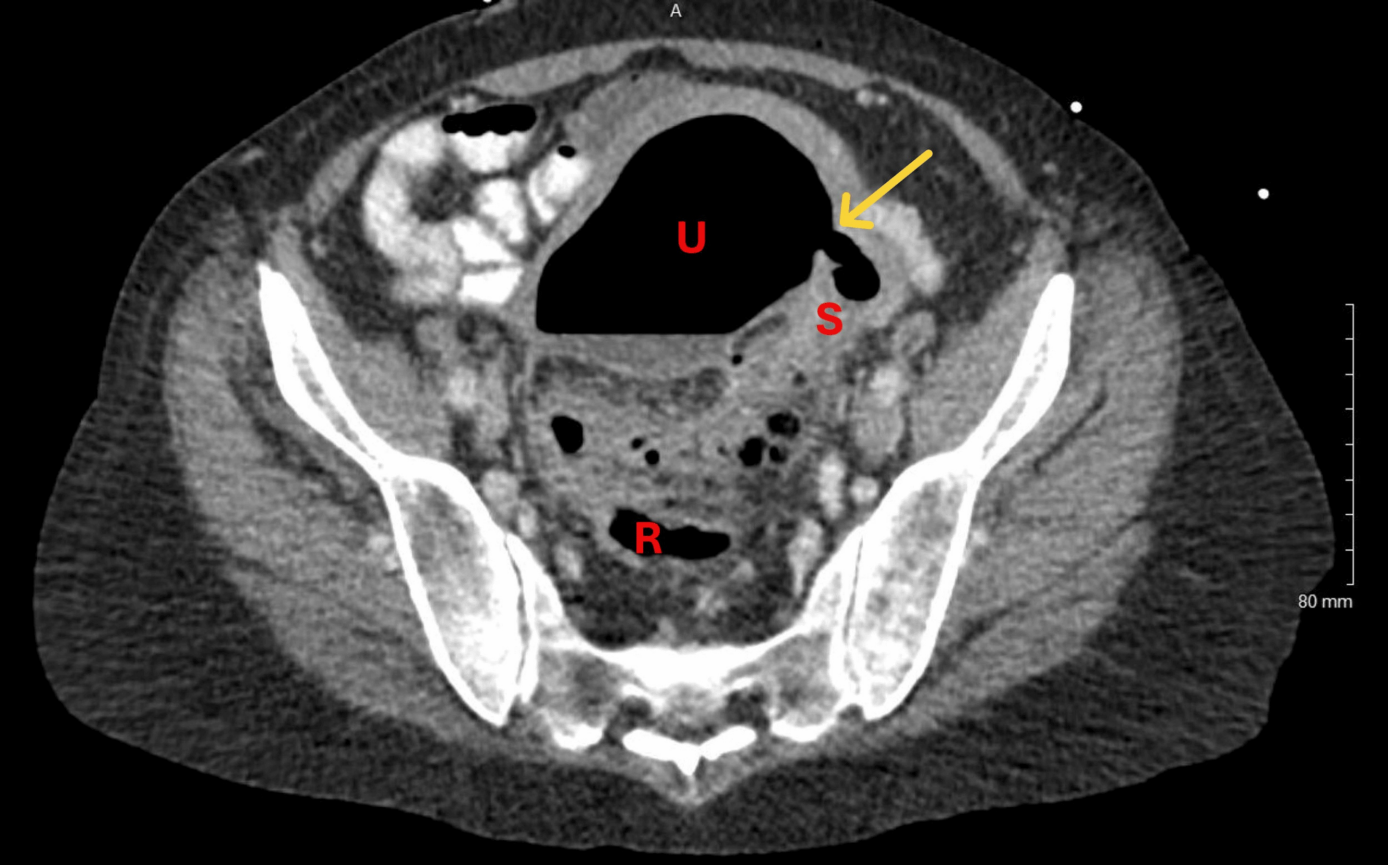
Contrast-enhanced computed tomography (CT) scan of the pelvis, axial view. The fistulous tract (yellow arrow) is shown between the uterus (U) and sigmoid colon (S). Rectum (R) is labeled for reference.
